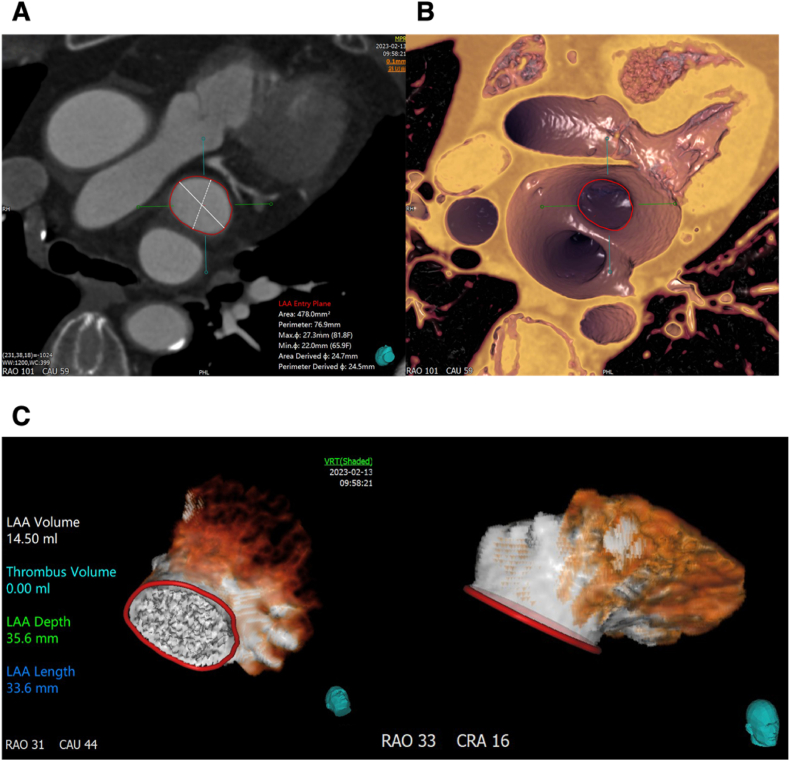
Pre-procedural Multidetector Computed Tomography Assessment of the Left Atrial Appendage (A, B) Multiplanar reconstructions show ostial area of 478 mm^2^ (maximum diameter 27 mm, minimum 22 mm). (C) Three dimensional volume rendered images (right anterior oblique 31°, caudal 44°) demonstrate an cauliflower shaped LAA volume of 14.5 mL, depth 35.6 mm, and length 33.6 mm.
